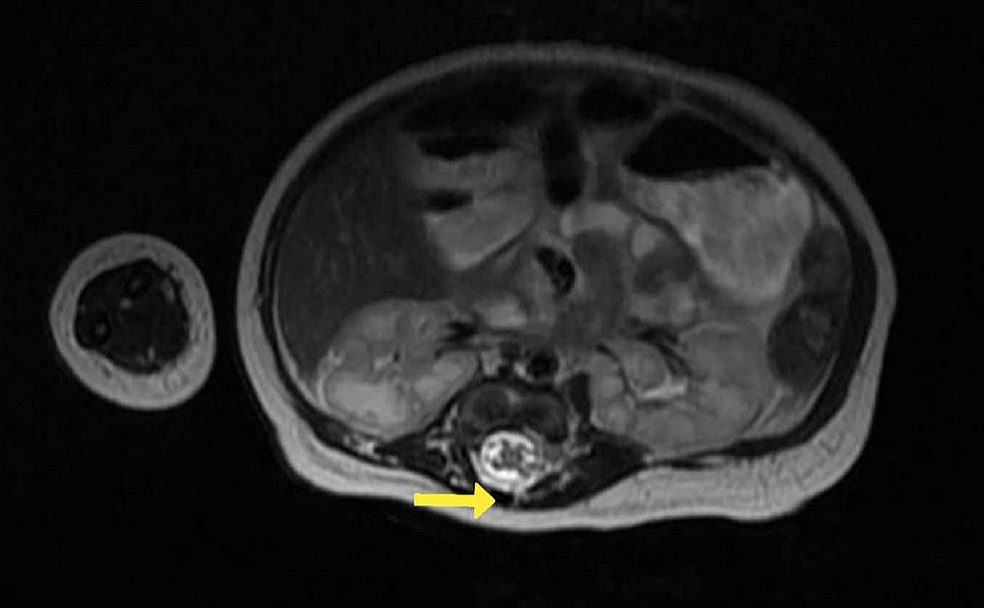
Axial T2 MRI image showing absent transverse process with overlying skin.
